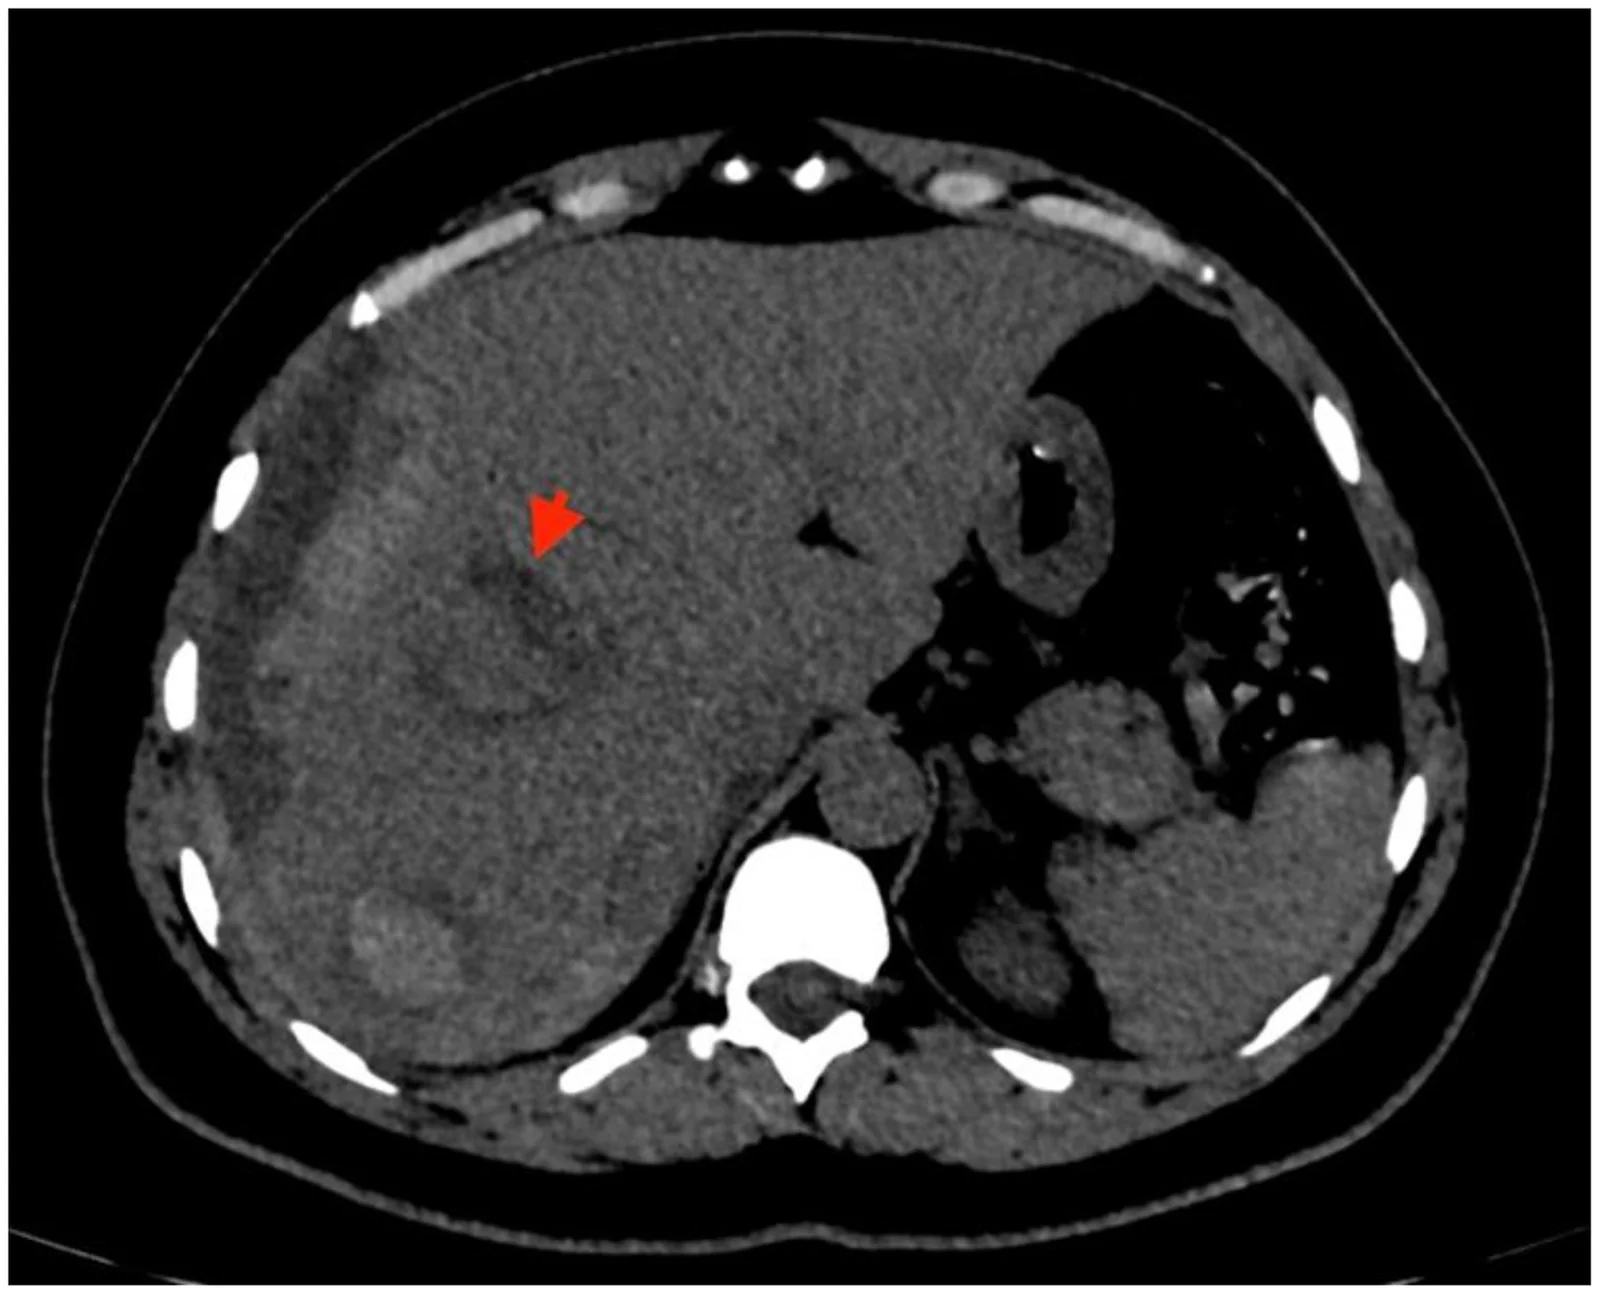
Unenhanced CT demonstrating a second distinct hemorrhagic hepatocellular adenoma in segment V. Axial unenhanced CT image showing a separate 3-cm lesion in hepatic segment V, containing spontaneously hyperattenuating areas consistent with intralesional hemorrhage (red arrowhead). This second distinct lesion confirms the multifocal nature of the hepatocellular adenomas.
